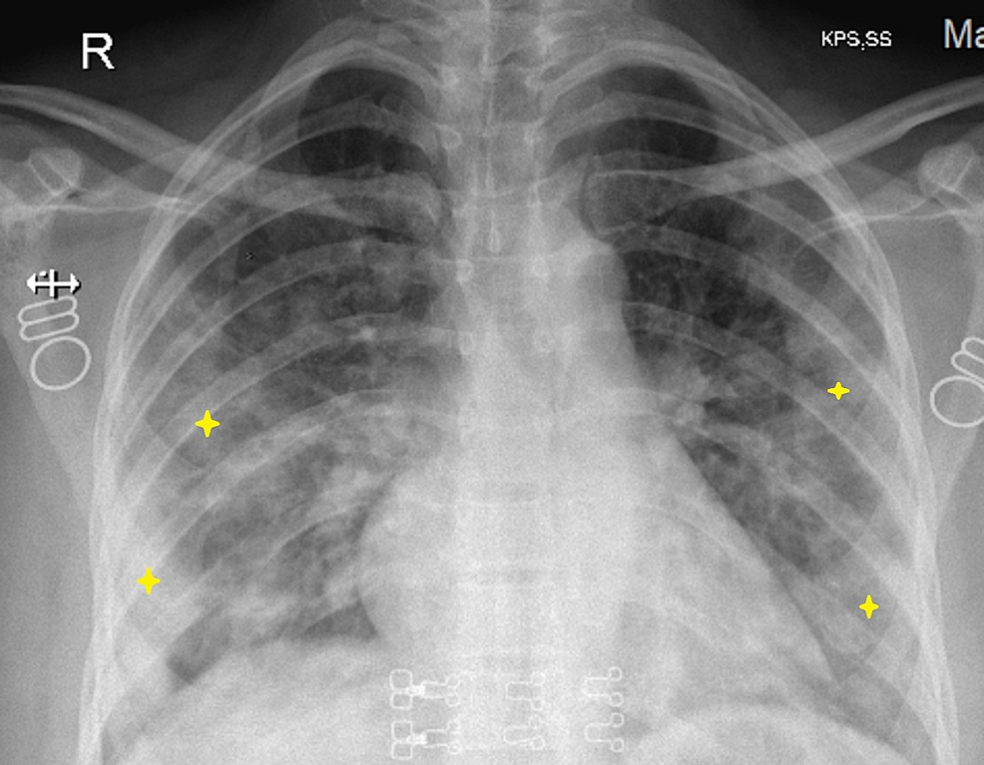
Chest X-ray showing diffuse radio-opacities bilaterally suggestive of COVID-19 pneumonia. COVID-19: coronavirus disease 2019
